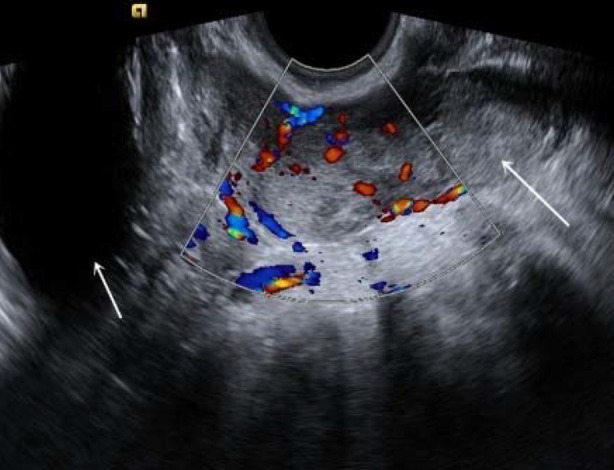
A patient with right type I PFTC (No. 9). The transvaginal color Doppler ultrasound showed the sausage-shaped hypoechoic solid mass in right adnexal region (long arrow), with rich blood flow signals and uterine fluid accumulation (short arrow) in flow imaging.
